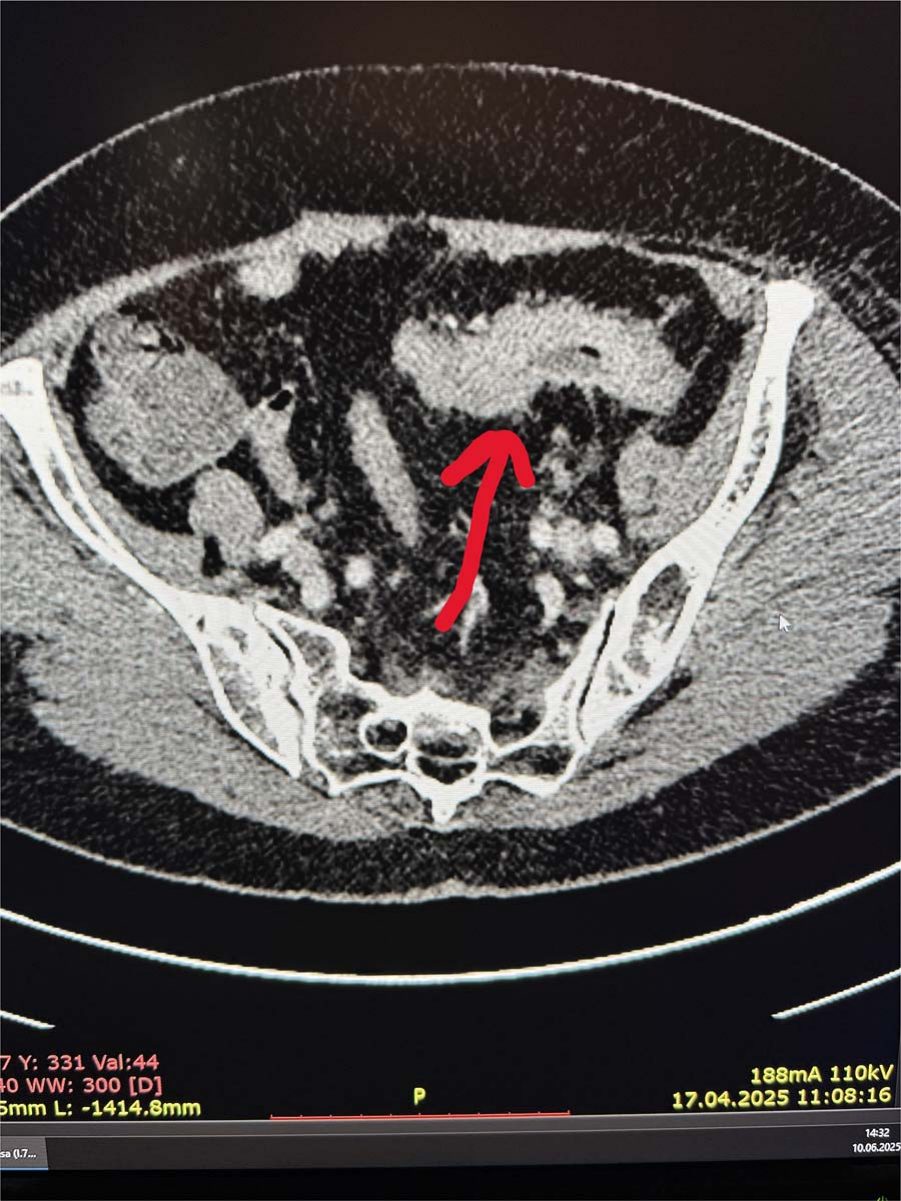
Sigmoid cancer on CT scan.
